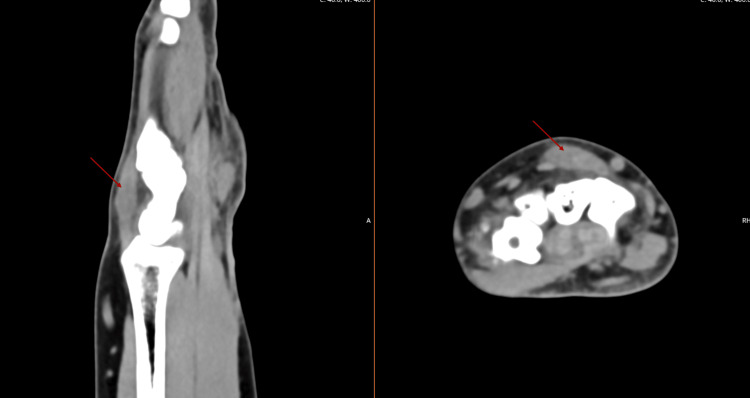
CT scan, intratendinous cyst (red arrow)
